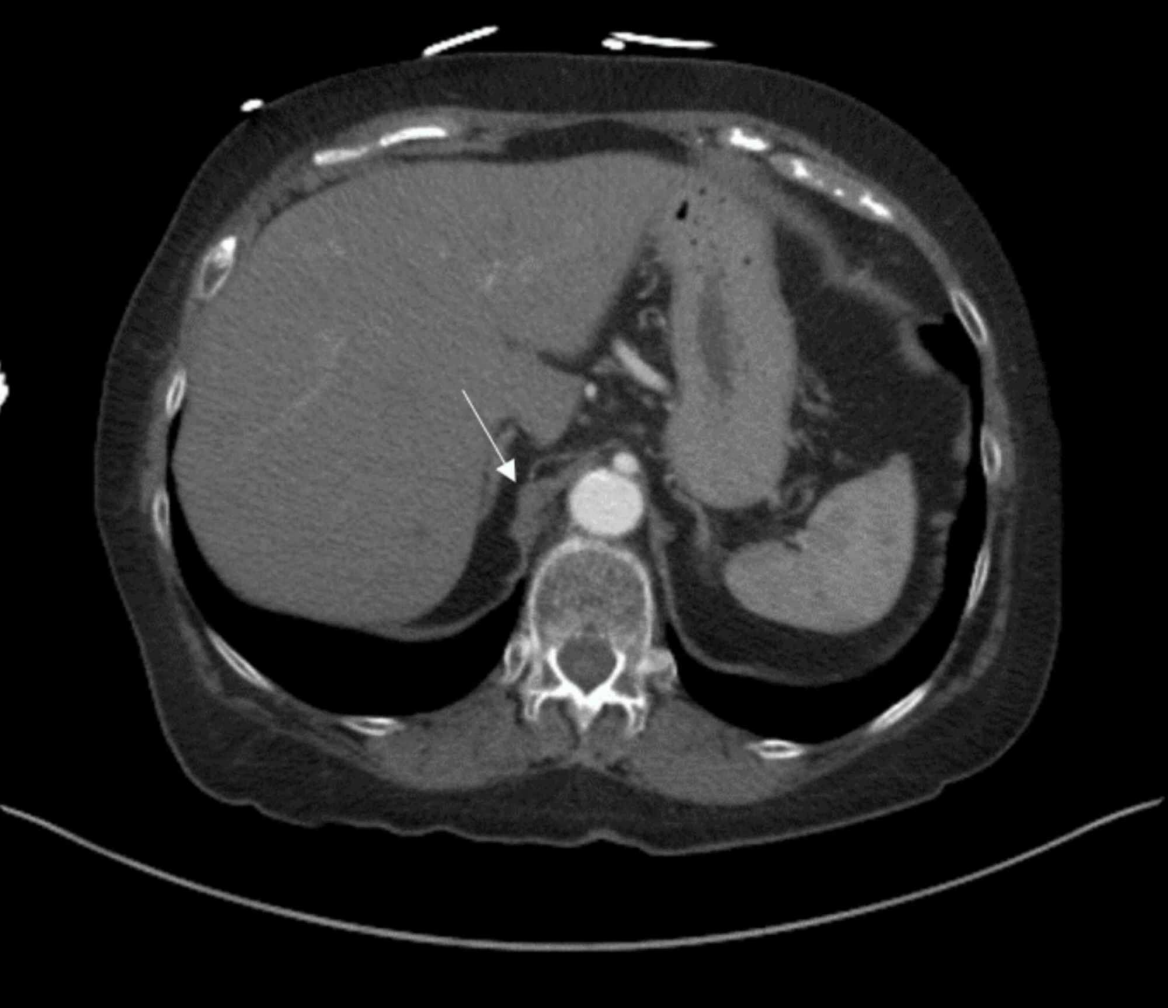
Axial contrast-enhanced CT image of abdomen/pelvis demonstrates the thickening of the median arcuate ligament on the right (arrow)
